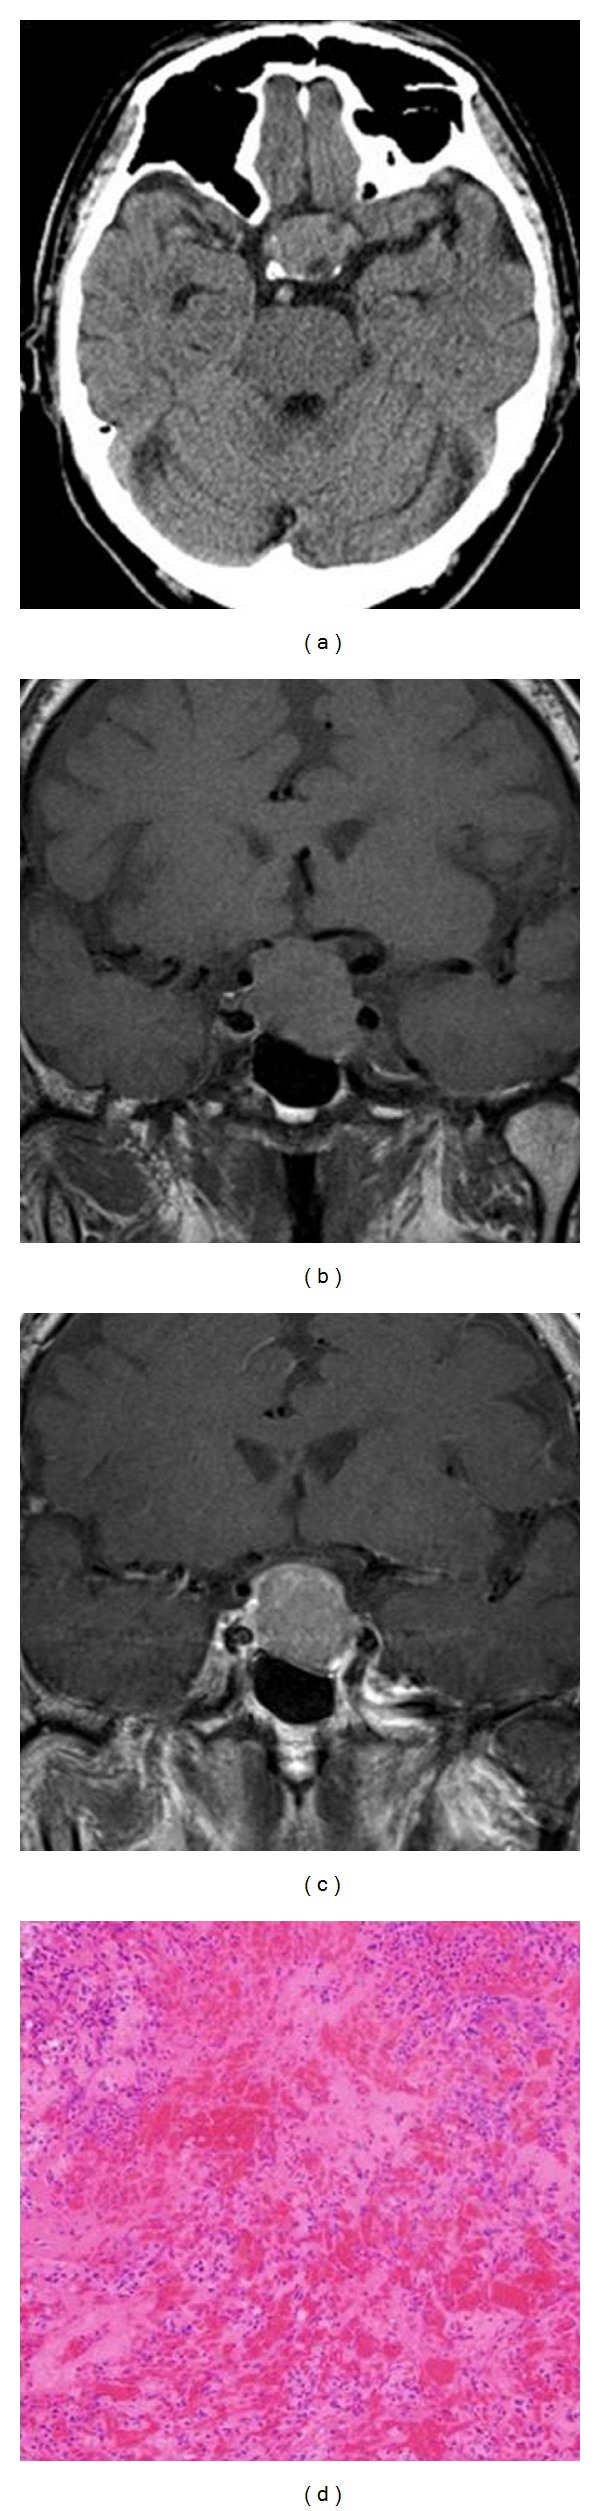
Case 2. CT scan performed 1 hour after onset demonstrates no evidence of intratumoral hemorrhage or acute enlargement of the tumor size (a), T1-weighted MRI performed after onset shows a pituitary tumor extending into the suprasellar cistern. There was no evidence of intratumoral hemorrhage or acute infarction (b), after onset gadolinium-enhanced T1-weighted MRI showing uniform enhancement of the pituitary tumor (c), and pathological examination revealed papillary-patterned adenoma with diffuse hemorrhage and necrosis (d).
